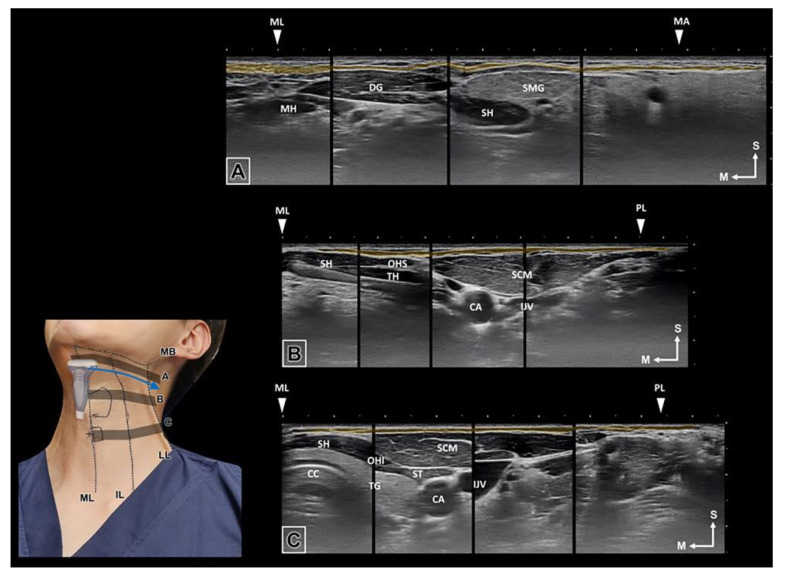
The ultrasonographic image of the platysma muscle was observed in blue arrow direction. The muscle lies from the midline to the vertical line from the mandibular angle and has been presented in the ultrasonographic image of three transverse planes: below the mandibular border (A), superior notch of the thyroid cartilage (B), and cricoid cartilage (C). (ML, midline; MH, Mylohyoid muscle; DG, Digastric muscle; SH, Sternohyoid muscle; SMG, Submandibular gland; MA, mandibular angle; CA, carotid artery; OHS, Omohyoid superior belly; OHI, Omohyoid inferior belly; IJV, Internal jugular vein; TH; Thyrohyiod muscle; TG; Thyroid gland; CC, Cricoid cartilage; IL, intermediate line; LL, lateral border of the lateral band).
